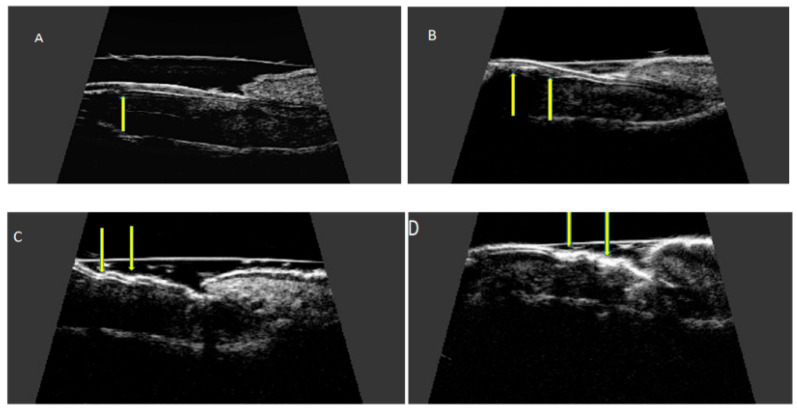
Ultrasonographic changes of the nail plate in nail psoriasis according to Wortsman classification. Longitudinal view. (A) focal hyperechoic involvement of the ventral plate—type I, (B) loss of the borders of the ventral plate—type II, (C) wavy plates—type III, and (D) loss of definition of both plates—type IV.
